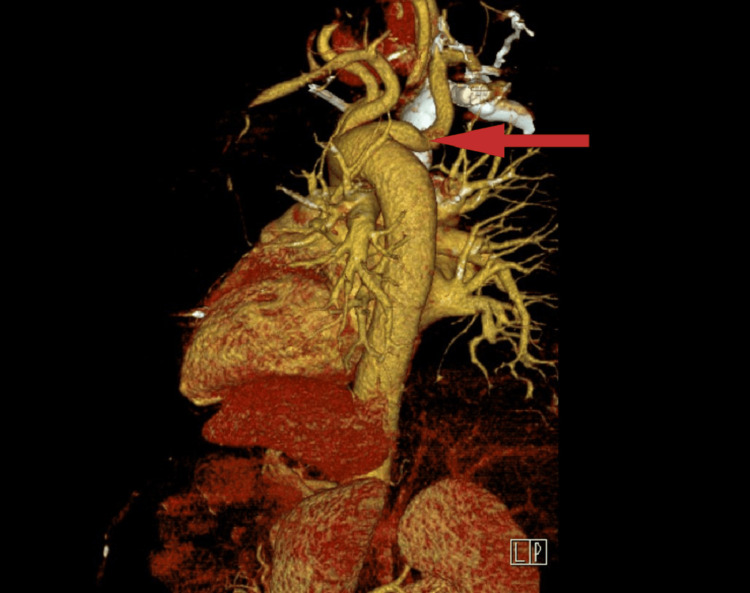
The 3D reconstruction of the chest CT angiography image visualizes an aberrant right subclavian artery (marked by the arrow) emerging from the aortic arch.
